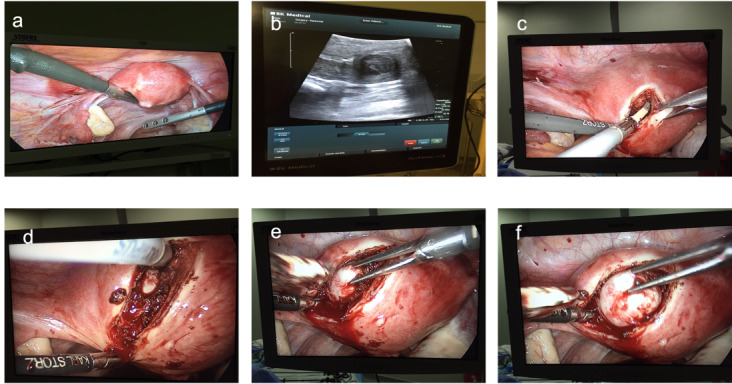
Deep intramural myoma indenting the uterine cavity removed laparoscopically under intraoperative ultrasound guidance: a. Locating the myoma with intraoperative ultrasound; b. Myoma visualized with intraoperative ultrasound; c. Placement of the serosal incision using the energy device; d. Identification of the cleavage plane of the myoma; e. Traction on the myoma using the endoscopic tenaculum; f. Removal of the myoma from the uterus.
